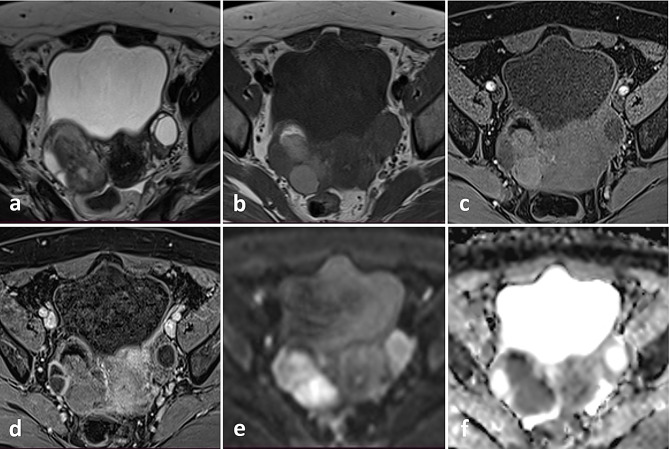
A right ovarian O-RADS MRI score 2 lesion. (a) Axial T2-WI shows a right-sided multiloculated adnexal mass of mixed signal intensity and left ovarian follicle (normal physiological observation). (b) Axial T1-WI shows high signal intensity locule at the right ovarian mass with a signal drop in T1 fat-saturated image (c) denoting lipid content. (d) Axial T1 contrast-enhanced image reveals a smooth wall, septal enhancement, and no enhanced solid tissue. (e) Axial DWI at b = 1000 revealed a high signal of the cystic component and hypointensity at the corresponding ADC image (f), denoting a restricted diffusion pattern with an ADC value of 0.907 × 10 − 3 mm2 /s. Pathology confirmed a right ovarian dermoid cyst
